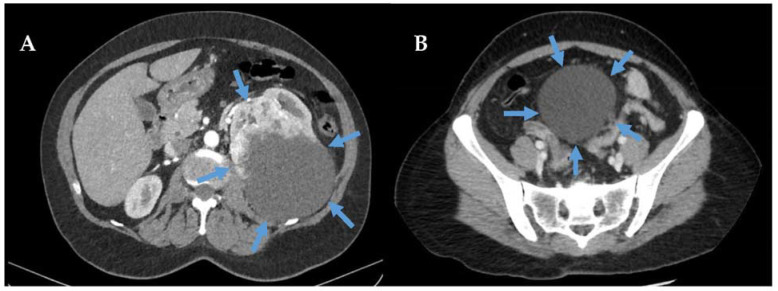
Primary retroperitoneal benign, AML arising from the kidney (blue arrows, Panel (A)) and primary retroperitoneal malignant PEComa-NOS arising from the rectosigmoid mesentery (blue arrows, Panel (B)). Both show similar CT characteristics, including density inconsistent with fat (−52 and −39 Hounsfield units, respectively) and well-circumscribed borders apart from the organ of origin (Kidney in Panel (A), and sigmoid in Panel (B)). While both AML and PEComa commonly arise from the kidney and perinephric fat, AML rarely, if ever, arises directly from the retroperitoneal tissues. Retroperitoneal core biopsy confirmed the diagnosis in both cases. The AML was managed with preoperative angioembolization and marginal excision of the left kidney and retroperitoneal tumour en bloc (R0 resection). The malignant PEComa-NOS was managed with radical resection, including rectosigmoid and low anterior resection, clearance of the retroperitoneal tissues above the aortoiliac axis, and right hemi-colectomy due to cecal abutment (R0 resection).
